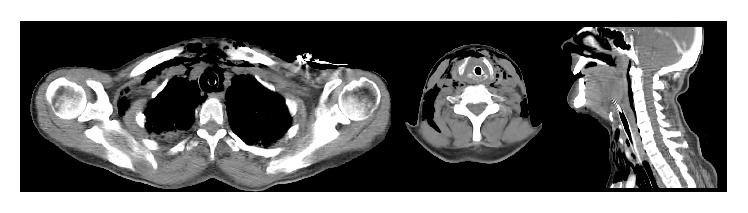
Neck and thorax CT-scan at admittance. It reveals anterior traumatic lesion of the larynx with severe emphysema of the cervical and supraclavicular area and displaced fracture of the thyroid cartilage. No laryngotracheal separation was observed.
